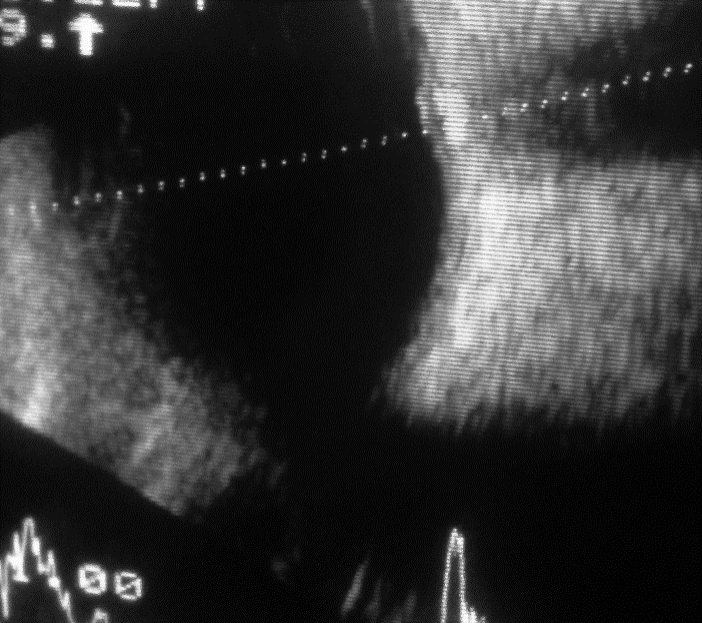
4 B-scan ultrasonography of both eyes showed optic disc elevation with the presence of buried drusen
